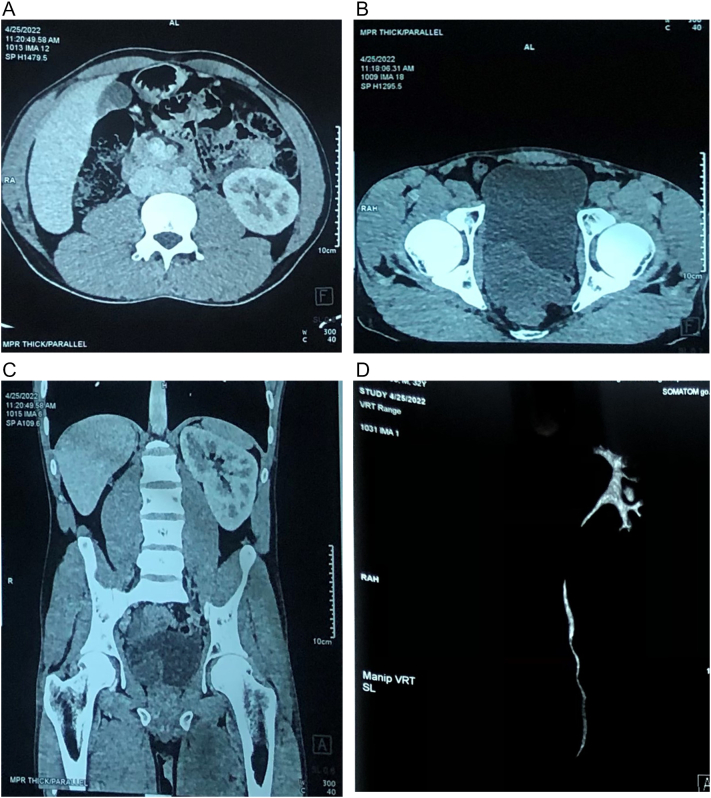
A: CT abdomen and pelvis: no evidence of the right renal unit. B: CT abdomen and pelvis: presence of a non-enhancing cystic lesion. C: CT abdomen and pelvis no evidence of the right renal unit with the presence of a non-enhancing cystic lesion. D: CT urogram: absent right kidney and right ureter.
